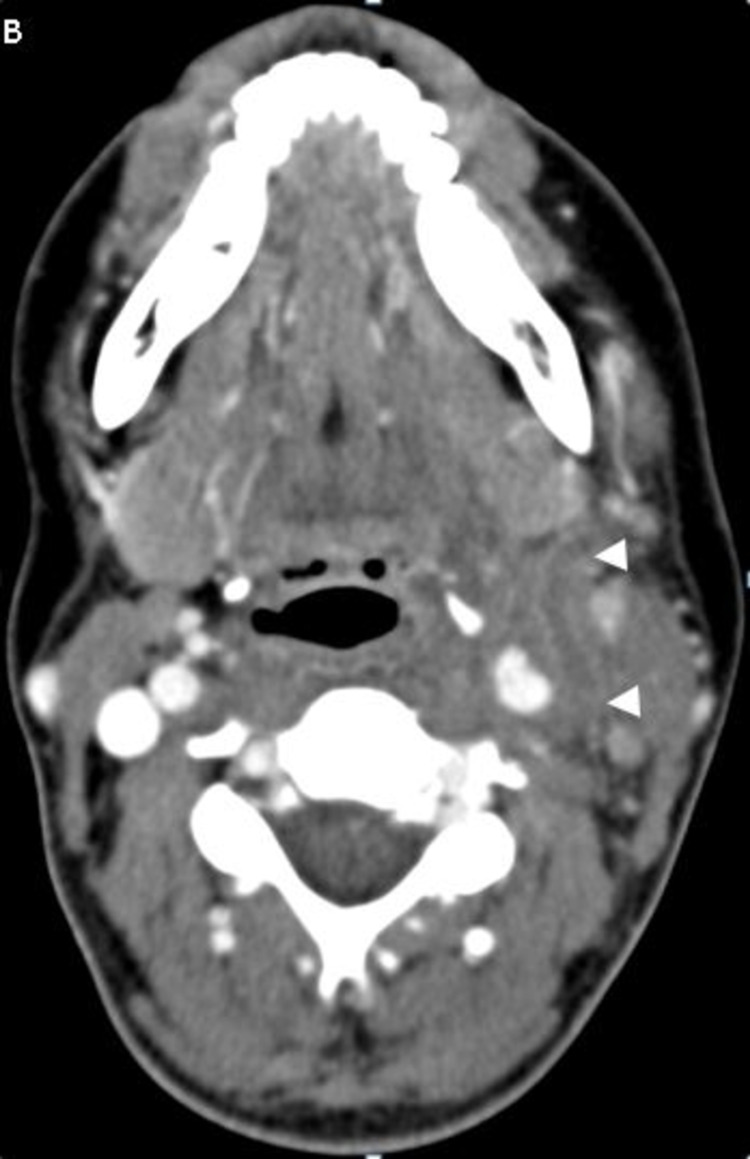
CT venogram of the neck CT image shows occlusion of the left internal jugular vein and left facial vein with surrounding fat stranding corresponding to areas of thrombophlebitis (white arrowheads) CT: computed tomography
